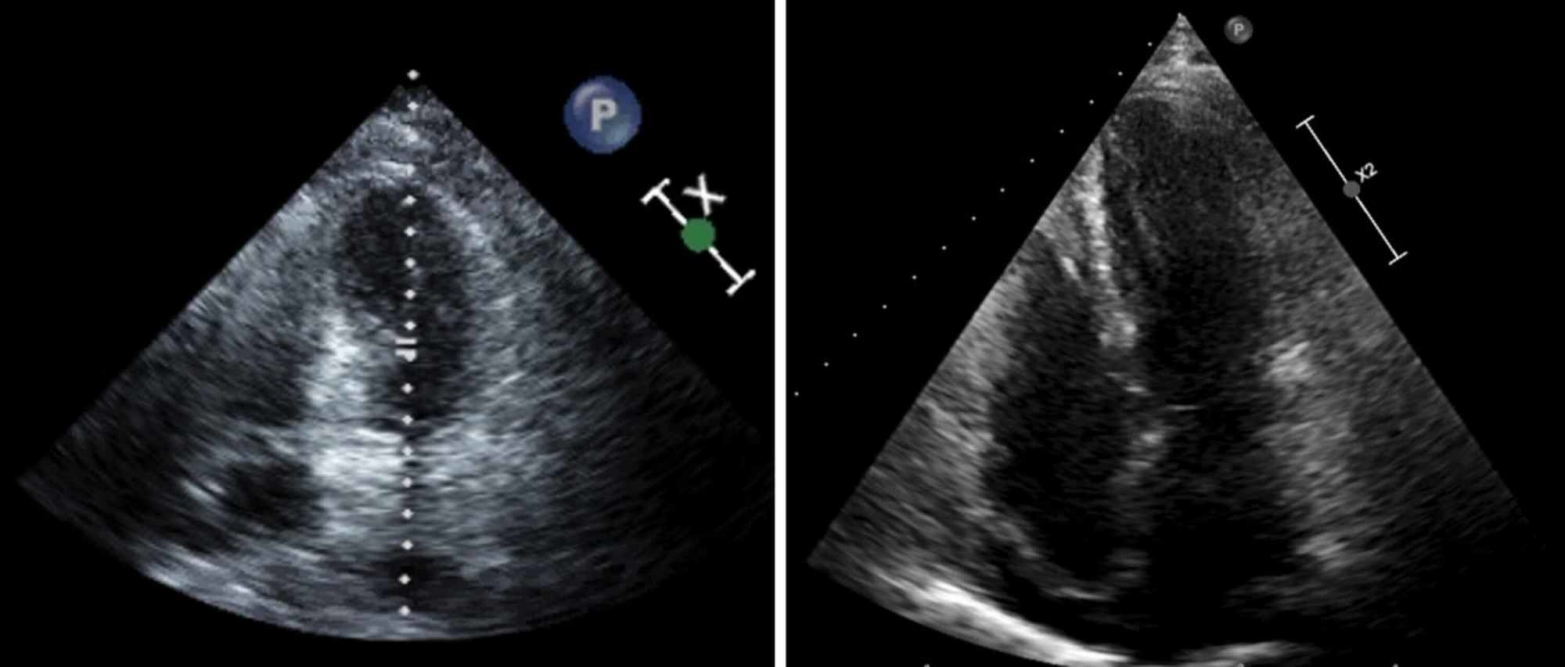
A. ECHO done on admission showing reduced systolic function. B. ECHO done one month later with EF of 60%-65%. ECHO: echocardiogram; EF: ejection fraction
